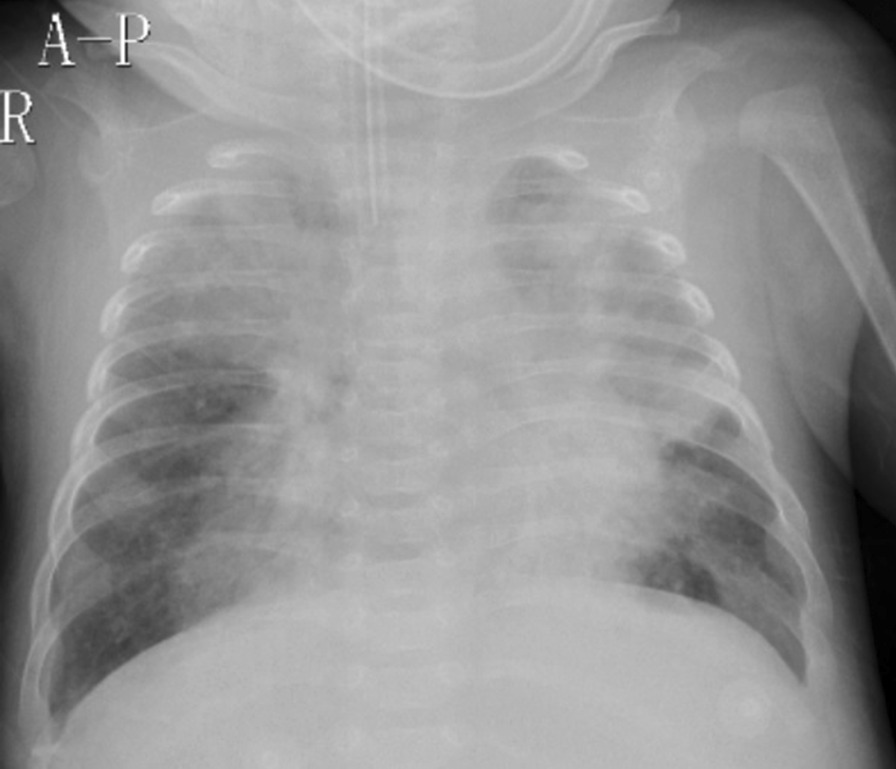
Chest radiograph showing bilateral diffuse infiltration and upper lungs are prominent indicative of acute respiratory distress syndrome
